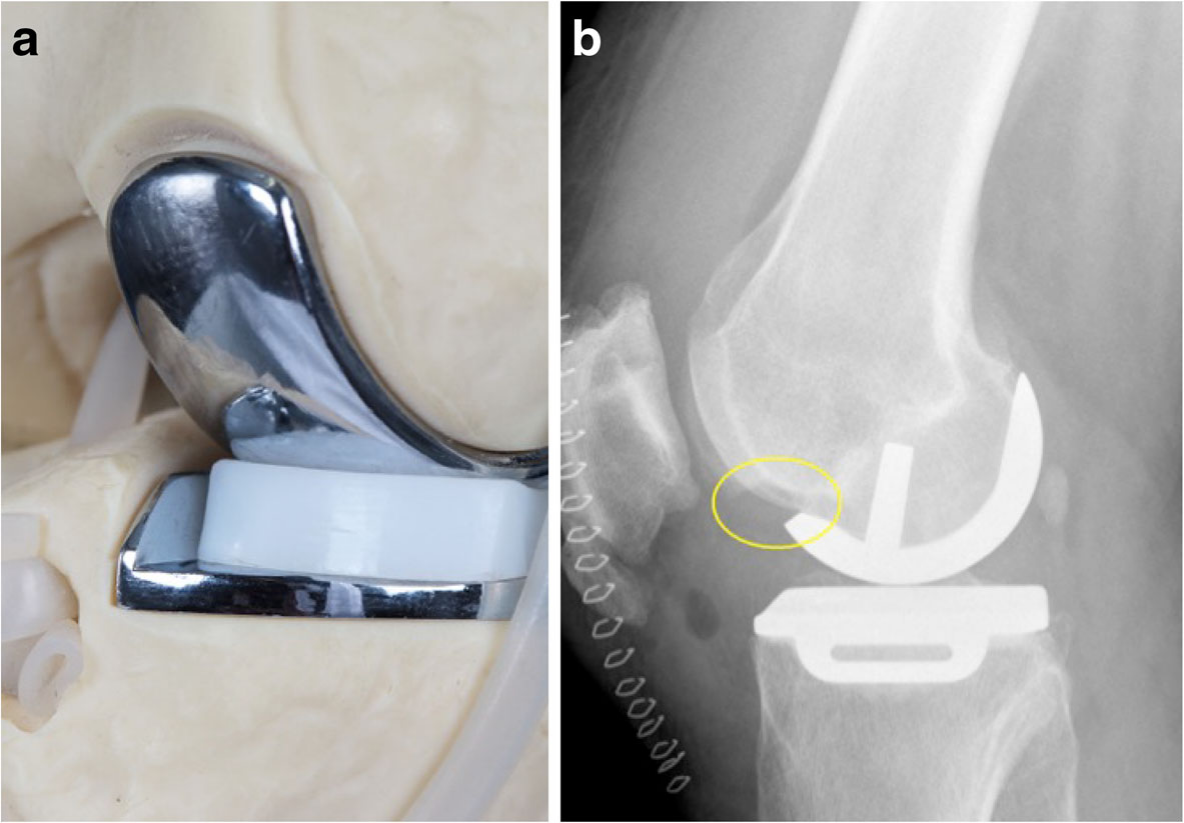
a. Saw bone model showing the anterior notch adjacent to the femoral implant (view from anteromedial). It is obligatory to create such a notch during mobile-bearing unicondylar knee arthroplasty b. Example of a rather large notch (10 mm) in one of our cases, as depicted in a lateral radiograph. The range of the notch size in our study population was 2–12 mm. The images were produced solely for this manuscript
